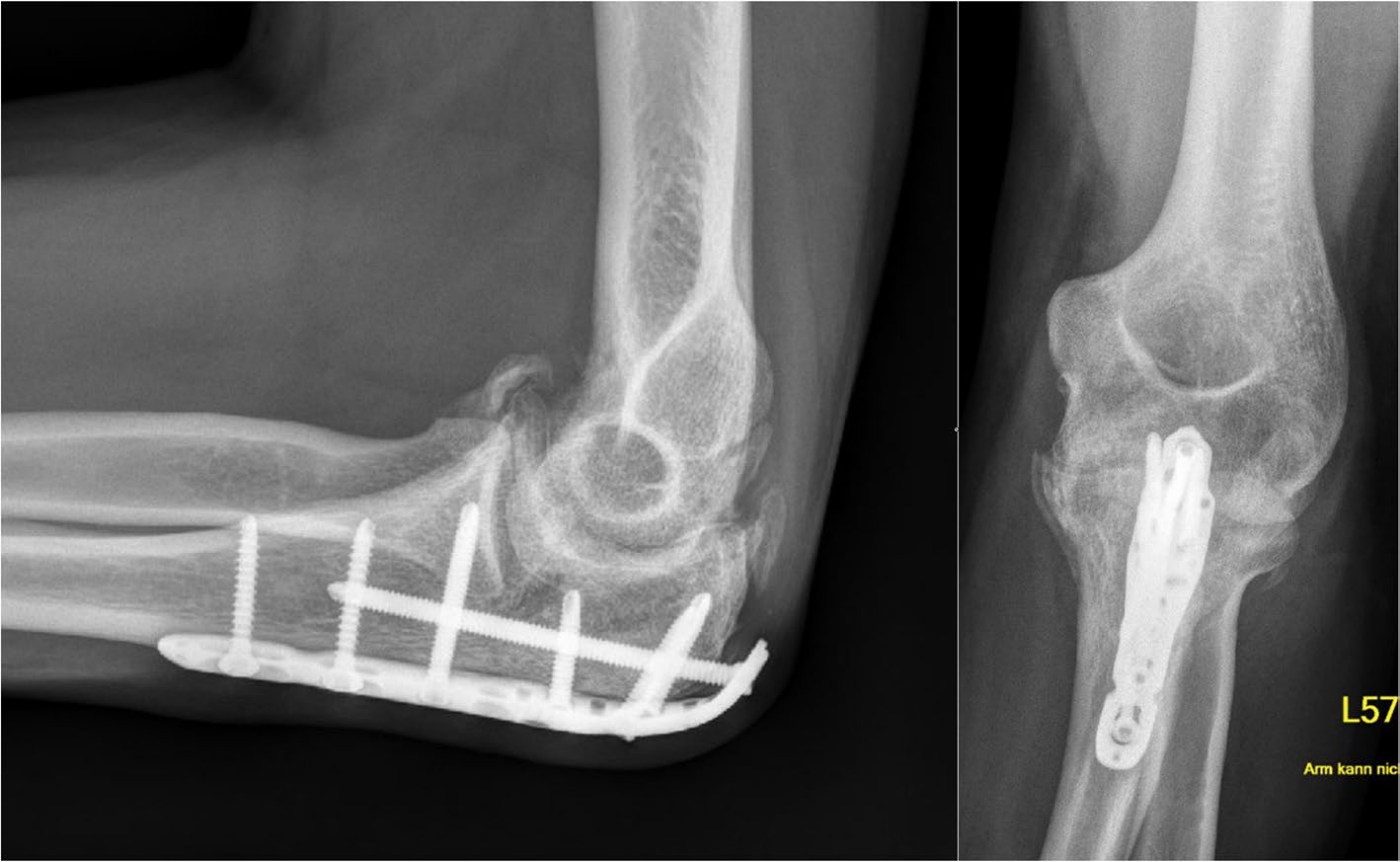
Radiographs in lateral and a.p. view of the left elbow of a 38 year old patient. One year after sustaining a Monteggia fracture and plate osteosynthesis of the olecranon
